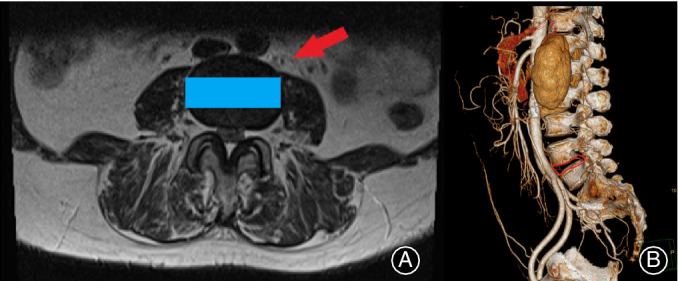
(A) Arrow indicates the route to the intervertebral disc and the rectangle shows the position where the cage is inserted. Cross‐section of the MRI determines if it is suitable to perform oblique lumbar interbody fusion (OLIF). (B) CTA is used to find whether there is abnormal vascular malformation to decrease the incidence of vascular injury.
